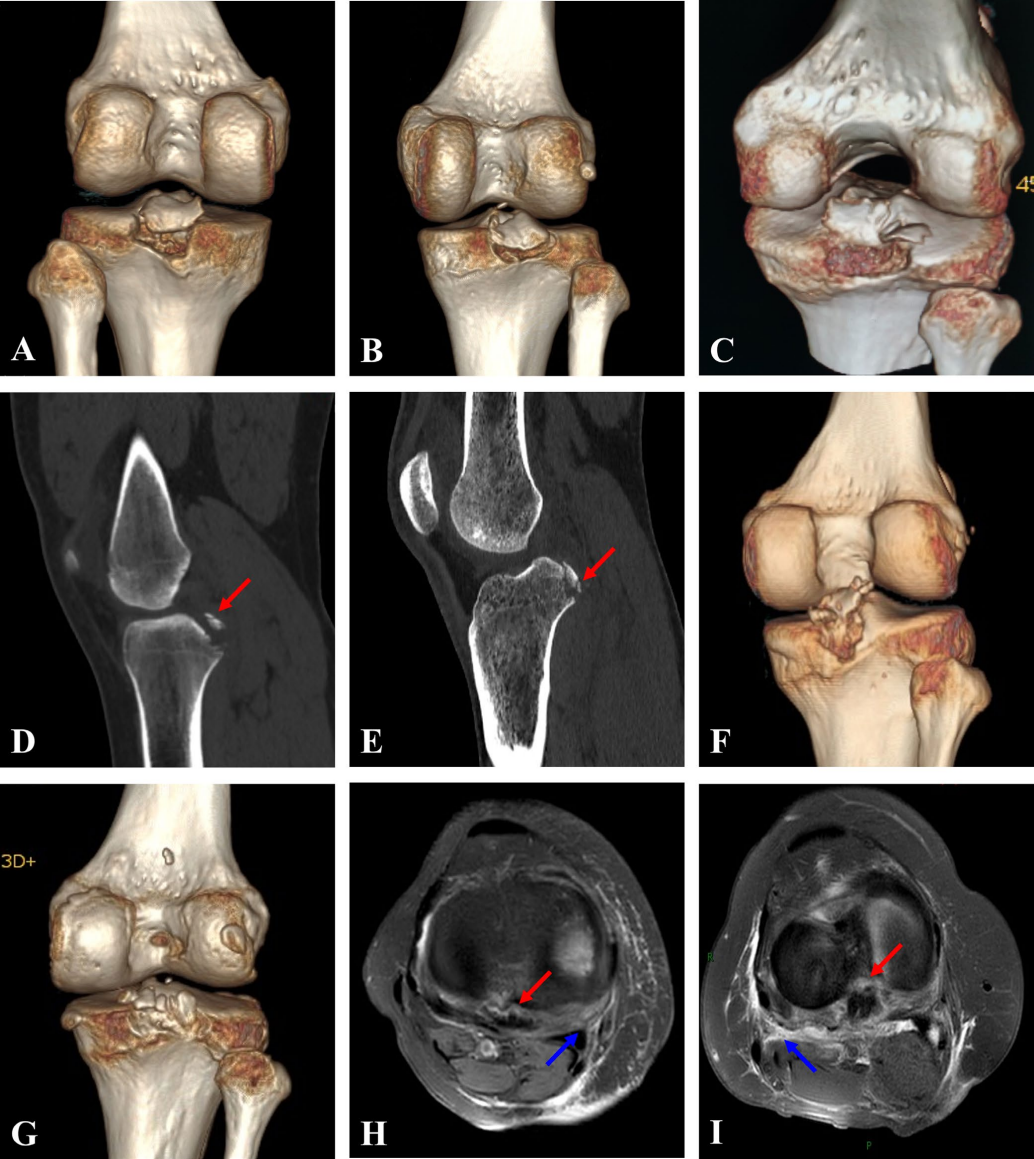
The CT (A:Type‐A, B:Type‐B, C:Type‐C, D:Type‐D, E:Type‐E, F:Type‐F, G:Type‐G) and the MRI (H:Type‐H, I:Type‐I) demonstrated that the types of posterior cruciate ligament avulsion fracture (red arrow) and the condition of soft tissue injuries (blue arrow).
